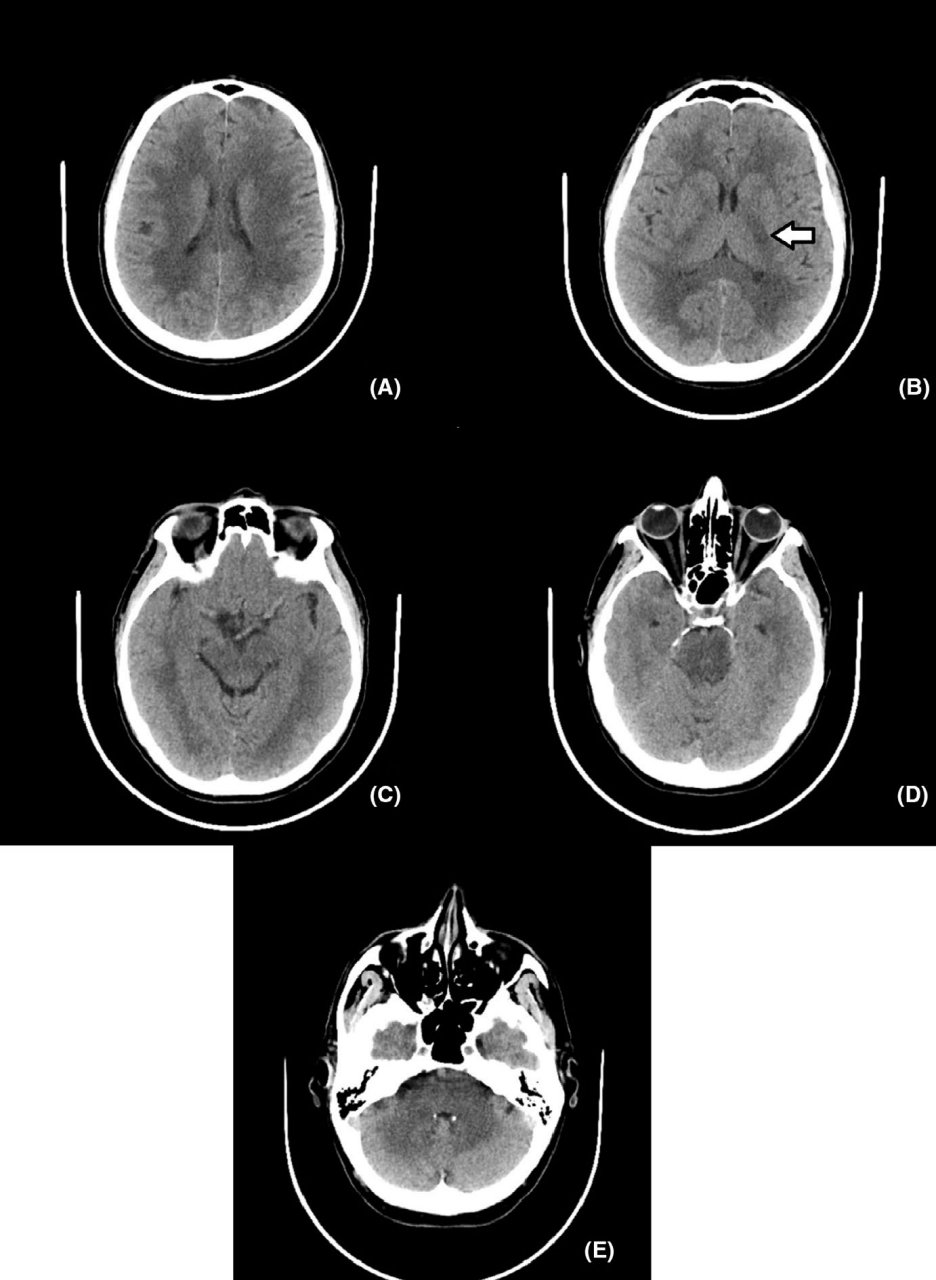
Computed tomography images showing diffuse white matter hypodensities in the centrum semiovale (A), inner capsule (B), midbrain (C), pons (D), and cerebellum and medulla oblongata (E). The arrow shows a small hypodense lesion in the posterior limb of the left internal capsule
